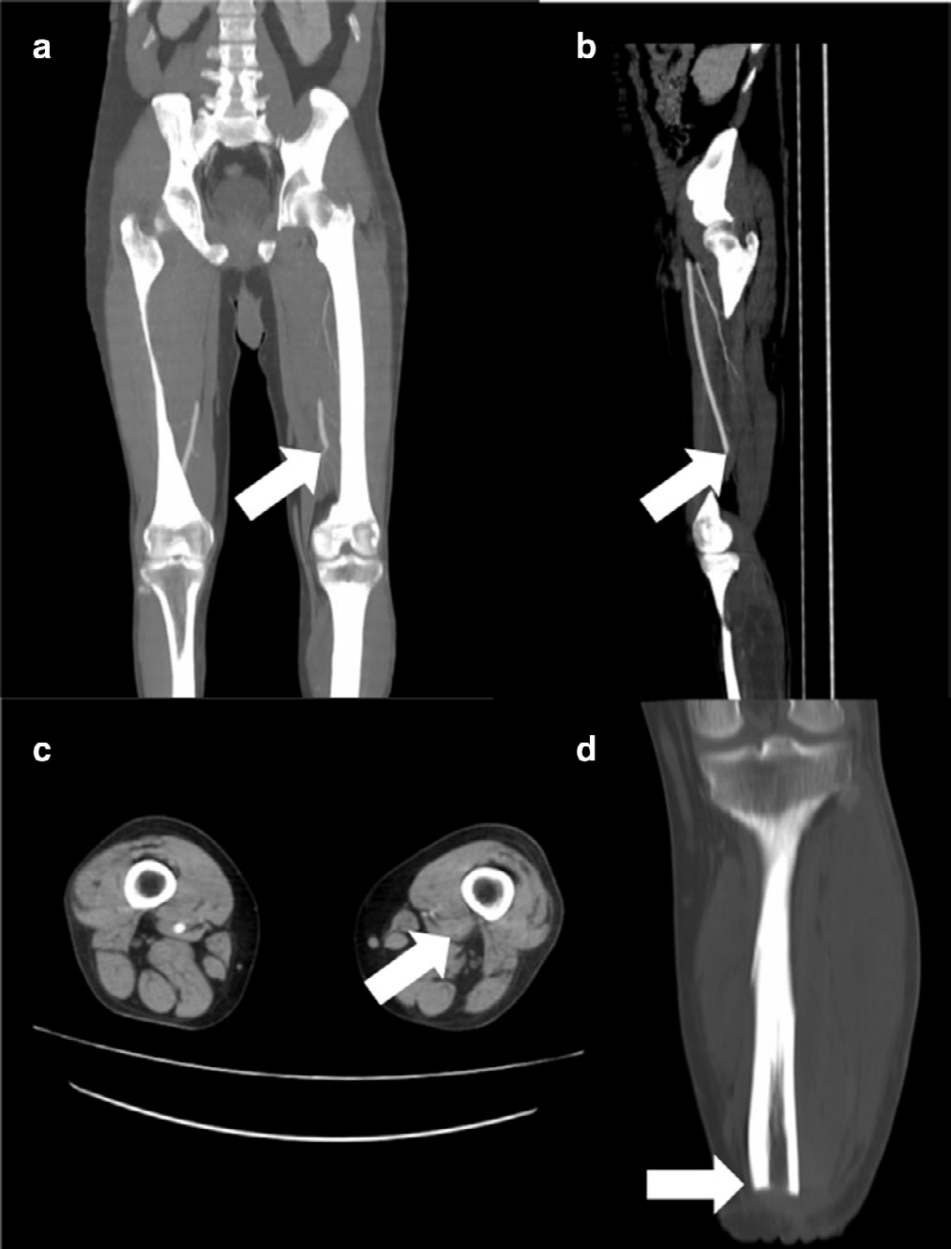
(A) Coronal, (B) sagittal and (C) axial post-contrast CT images showing absence of the intravascular contrast in the left arterial femoral-popliteal segment compatible with thrombosis. (D) Coronal CT image showing post-treatment changes after amputation.
